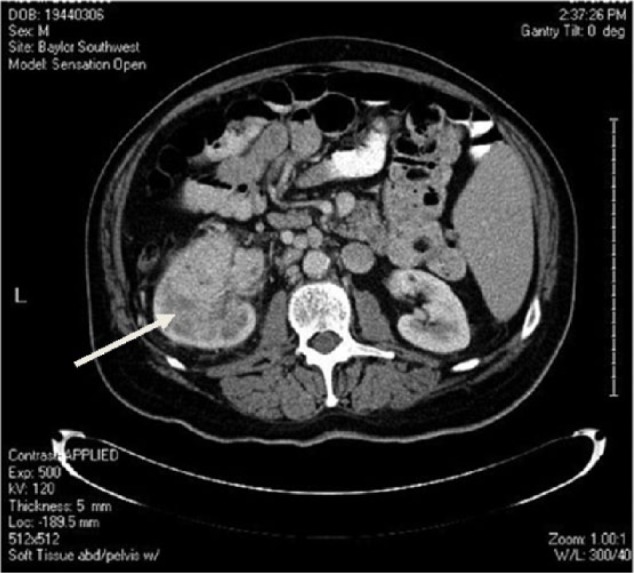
June 2008, CT scan showing a large hyperdense mass (arrow) occupying the mid
